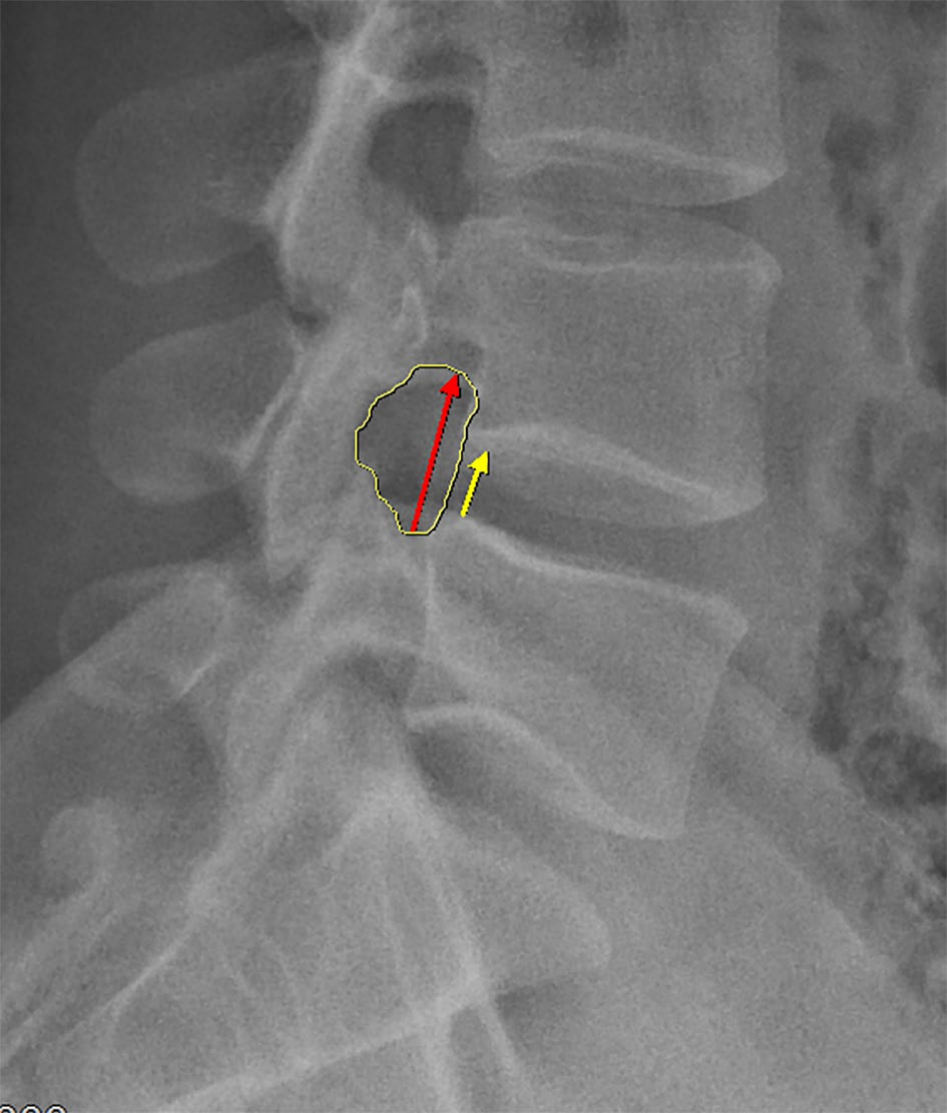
Overview of the radiographic parameters of interest. Yellow arrow indicated PISH; red arrow indicated IFH; yellow area indicated IFA.
